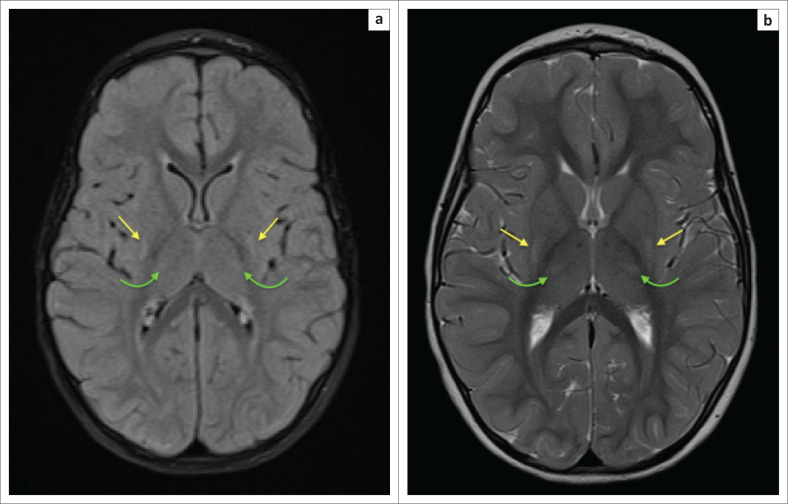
A 2-year-old female child with dystonic cerebral palsy. (a) An axial FLAIR sequence image and (b) an axial T2-weighted image demonstrating even more subtle signal abnormalities at the dorsal putamina (yellow arrows) and ventral thalami (green arrows). These changes can be very difficult to detect and may be omitted by the unsuspecting reporter.
